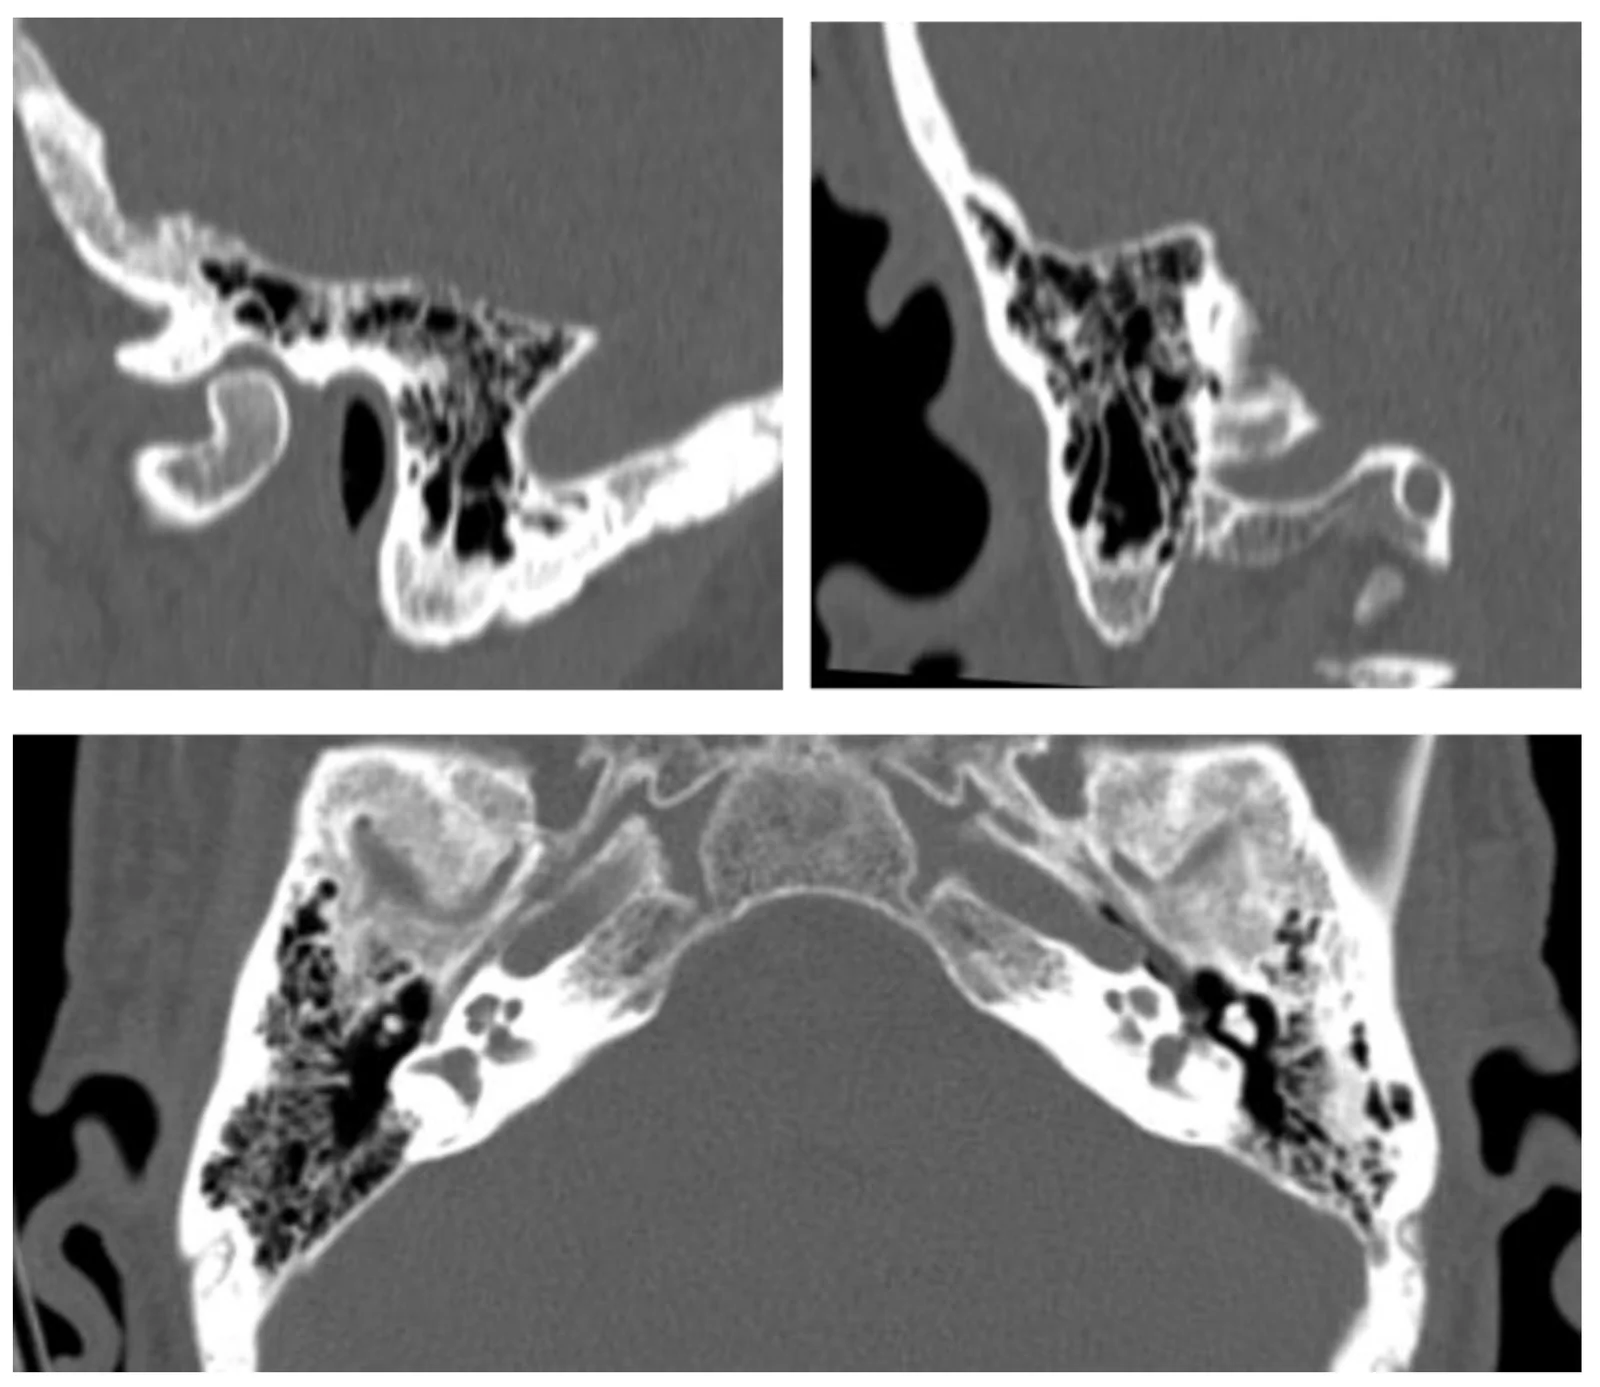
Mastoid and temporal bone CT dataset in sagittal, coronal, and axial planes.
